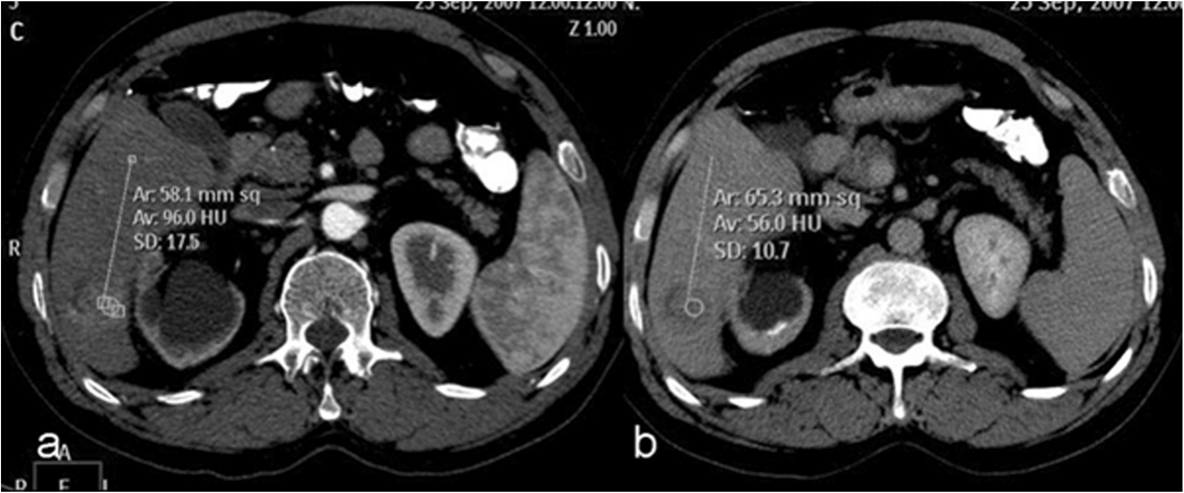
Measurements of computed tomography (CT) attenuations (in Hounsfield units) of a hypervascular hepatocellular carcinoma on arterial (a) and delayed (b) phase CT images.
